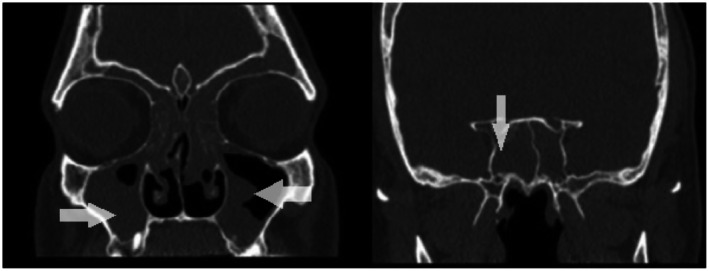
Coronal paranasal sinus CT scan, soft‐tissue window, Day 0. The image shows near‐total bilateral maxillary and complete bilateral sphenoid sinus involvement. Thick white arrows highlight the regions of opacification before treatment.
